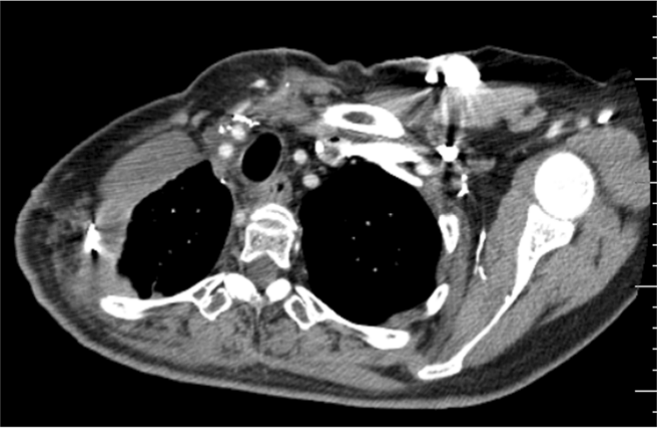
Coronal CT taken three years after surgery. There is no evidence of recurrence or invasion of malignancy.
